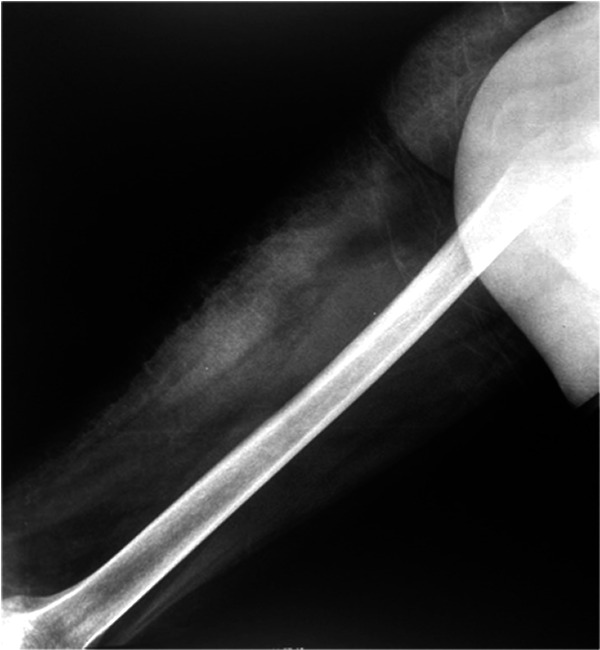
Conventional radiography of the left femur showing a thickening of the soft tissues of the middle and posterior region of the thigh with integrity of the bone.
